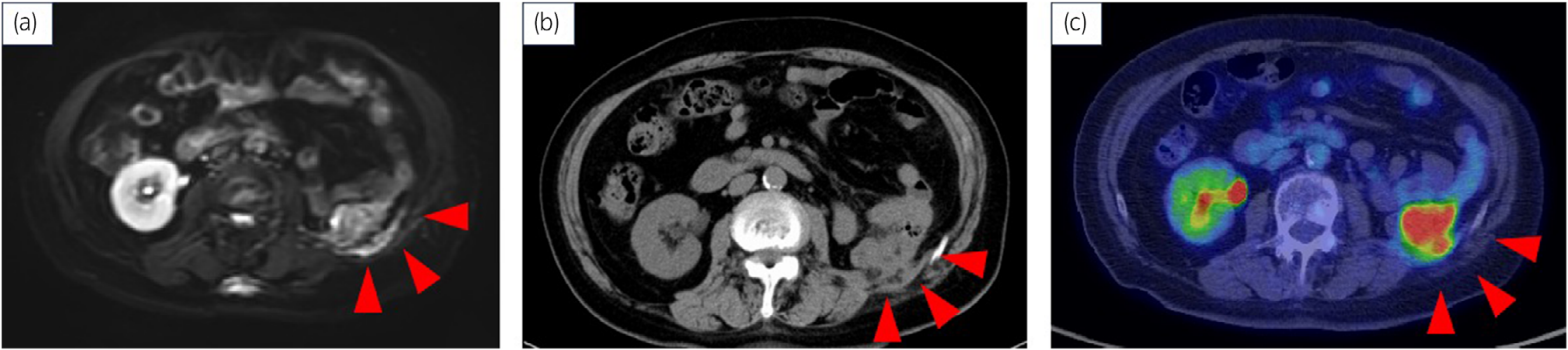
Post‐recurrence imaging. (a) MRCP showed a peritoneal lesion (diffusion weighted imaging). (b, c) PET‐CT showing FDG uptake in the peritoneal lesions.
